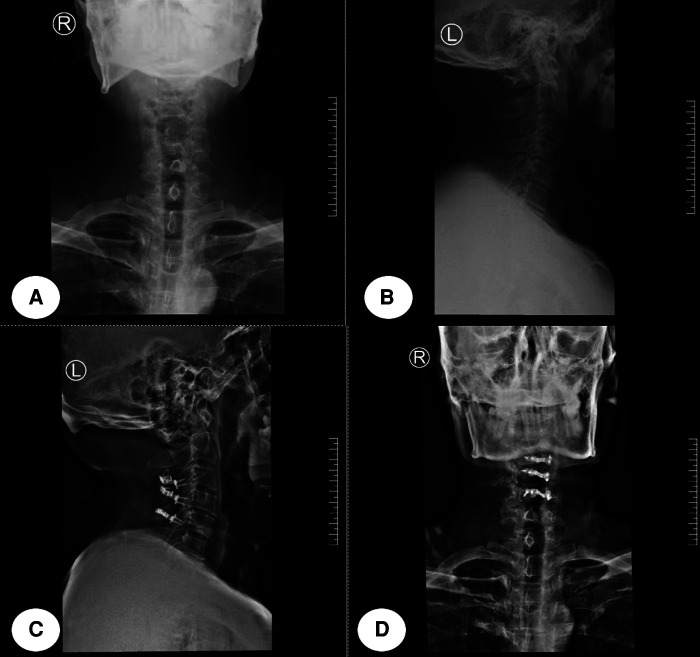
This was a typical case. A 45-year-old man was diagnosed with CSM. The VAS score was 1 before cervical posterior laminoplasty and the postoperative VAS turned to 4. [(A) and (B) showed the preoperative cervical x-rays both in anterior–posterior and lateral positions; (C) and (D) showed the postoperative cervical x-rays].
